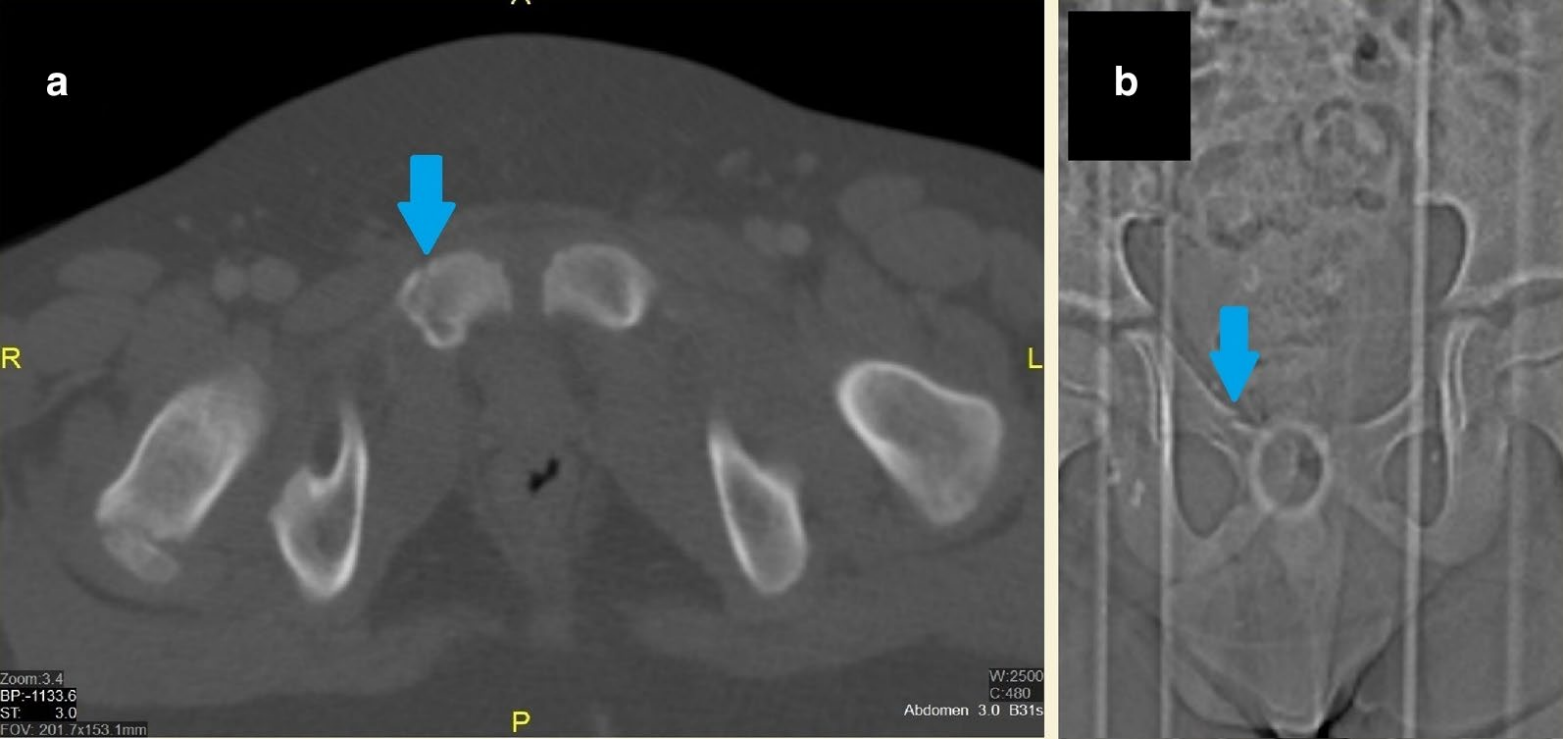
Scout neglect bias. An abdominal CECT of a male patient was performed to evaluate solid organ injury after a motor vehicle accident. A right pubic fracture (blue arrows) was masked in routine abdominal CT images of 3 mm slice thickness with a soft filter (a). However, it was easily seen in the scout image without any doubt (b)
